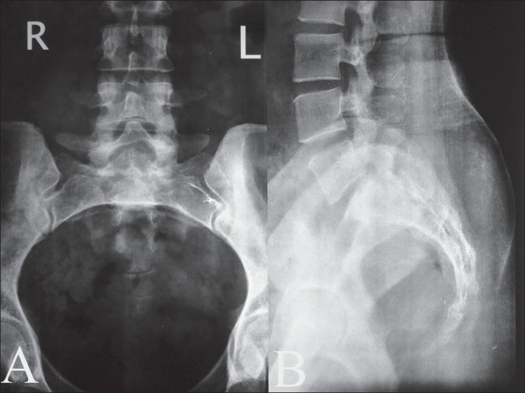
X-ray of lumbosacral spine antero posterior (A) and lateral (B) view of the patient showing no obvious bony changes
